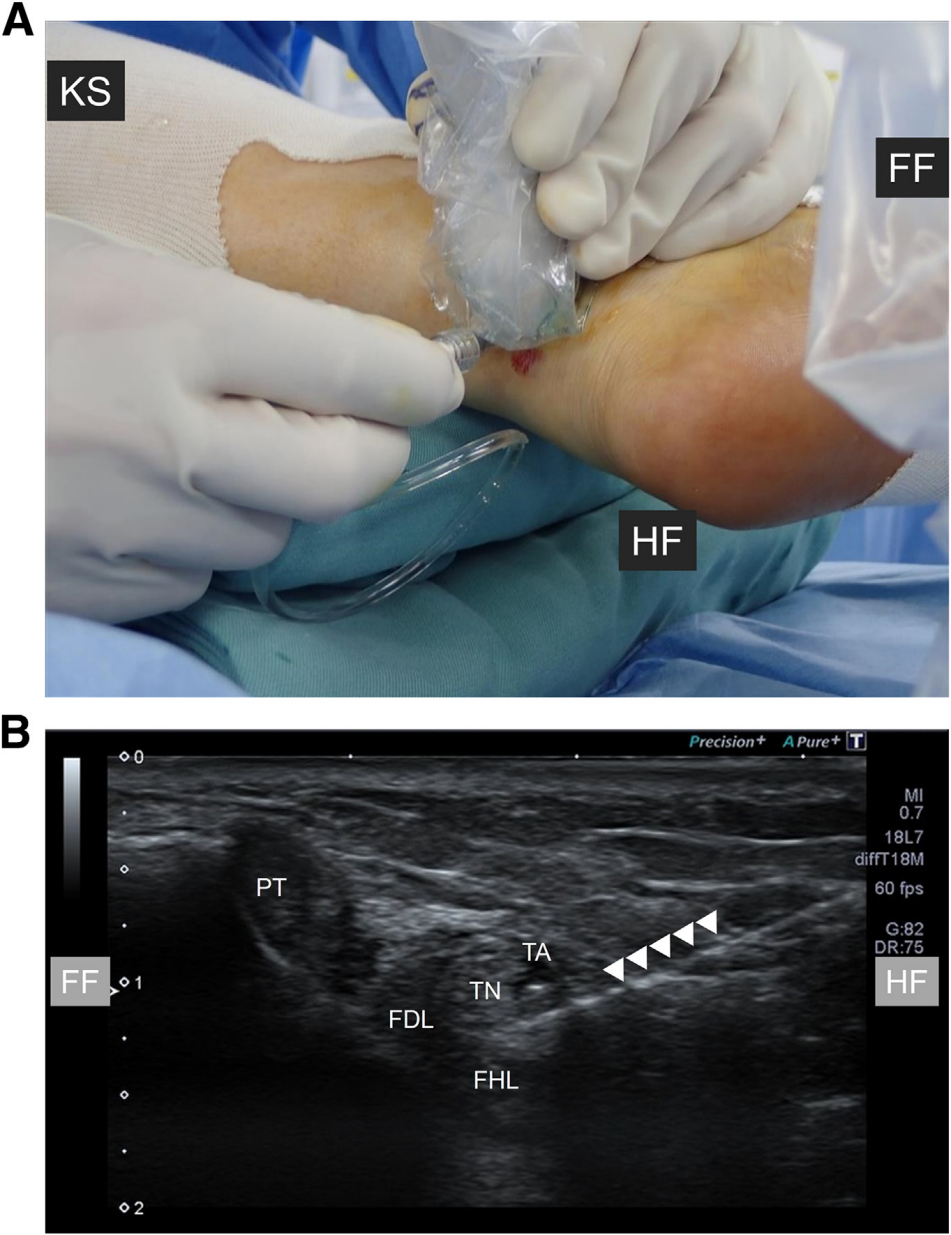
Injection of 1% lidocaine into the left foot to separate neurovascular bundle from flexor hallucis longus (FHL) tendon under ultrasonographic guidance. (A) The needle is inserted from the medial side of the Achilles tendon. The patient remains in the supine figure-of-4 position. (B) An ultrasonographic image shows the needle tip (arrowheads) located between the tibial artery (TA), tibial nerve (TN), and FHL tendon, confirming a safe injection space. (FDL, flexor digitorum longus tendon; FF, forefoot side; HF, hindfoot side; KS, knee side; PT, posterior tibial tendon.)
